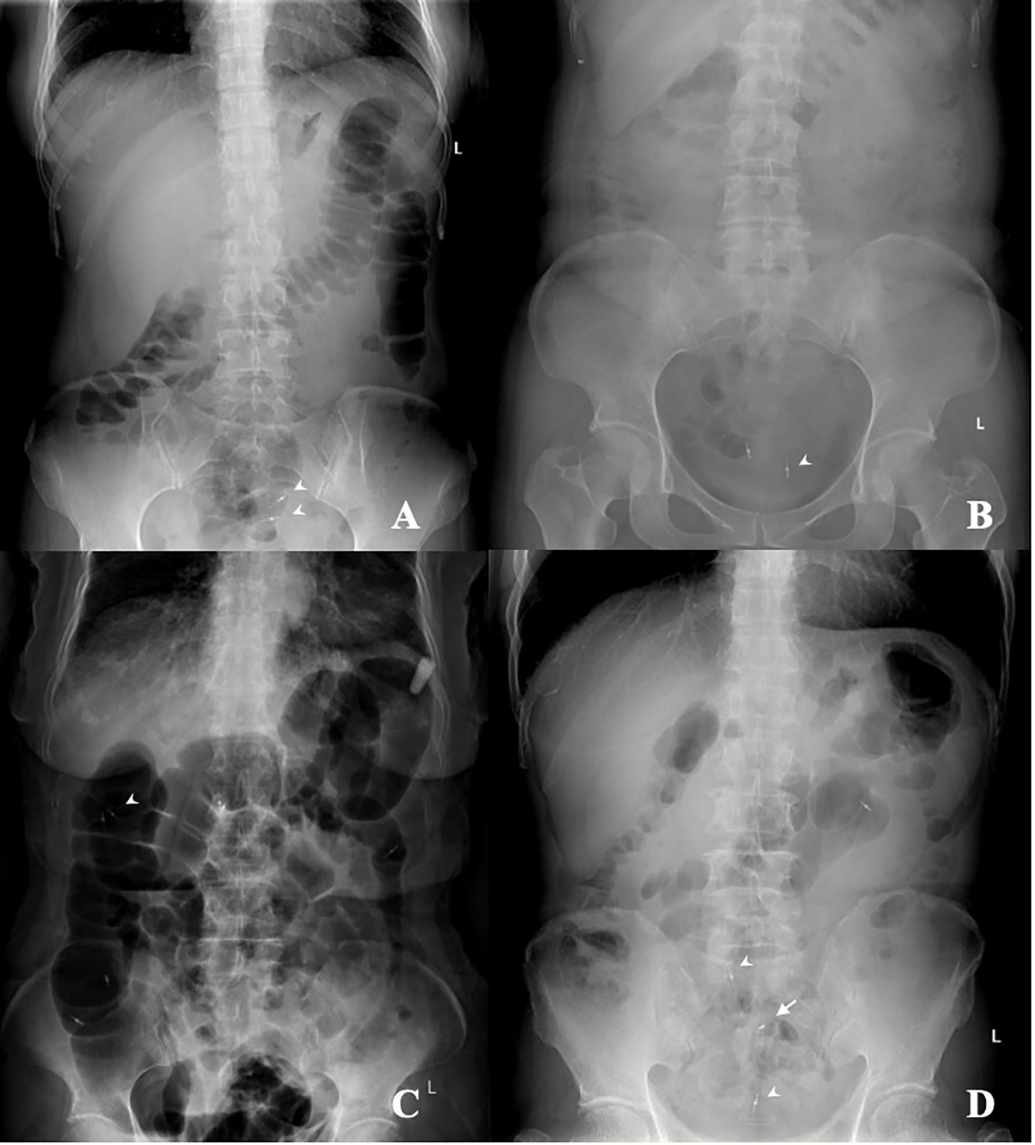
The supine abdominal plain film after clip placement for tumor localization. (A) The appropriately air-inflated colon and the clips (arrowhead) near the tumor in the upper rectum. (B) The poorly air-inflated colon and the clips (arrowhead) near the tumor in the rectosigmoid junction. (C) The over air-inflated colon and the overlapped lumen of the ascending colon and transverse colon, and the clips (arrowhead) failed to localize the tumor in the transverse colon. (D) Several clips in the sigmoid colon, with two clips (arrowhead) placed after polyp removal and one clip (arrow) placed near the tumor, which would create confusion.
